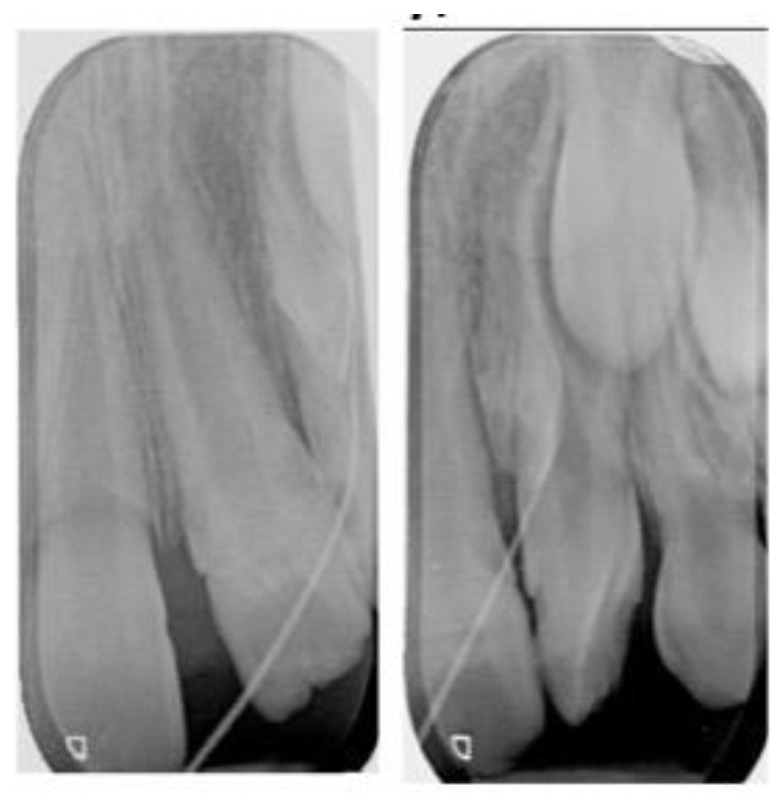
Periapical radiographs at initial assessment taken at different angles to locate the “extra tooth” with a Gutta-Percha point to trace the sinus tract.
